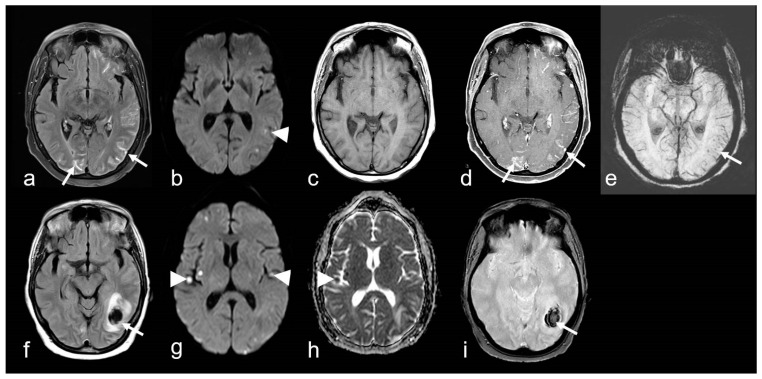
A 68-year-old woman suffering from progressive dizziness and visual blurring for several weeks (upper row) and acute deterioration (lower row) due to cerebral amyloid angiopathy-related inflammation (CAA-ri) with associated vasculitis (amyloid-beta-related angiitis, ABRA). (a–e): Multifocal hyperintense sulcal effusions ((a), arrow; fluid-attenuated inversion recovery (FLAIR)), focal small lesions with restricted diffusion temporo-parietal left ((b), arrowhead; diffusion-weighted imaging (DWI, b = 1000 s/mm^2^)), distinct multifocal leptomeningeal enhancement ((d), arrow; (c,d): T1 WI before (c) and after (d) contrast agent application); (e): multiple microbleeds (arrow, susceptibility-weighted imaging (SWI)). (f–i): Subacute lobar intracerebral hemorrhage (ICH; (f), arrow); (g,h): new cortical / subcortical infarcts ((g,h): arrowhead; DWI, b = 1000 s/mm^2^, apparent diffusion coefficient (ADC) map); (g): T2* WI demonstrating inhomogeneous signal loss (arrow); MRI 1.5 T Intera, Philips Healthcare.
